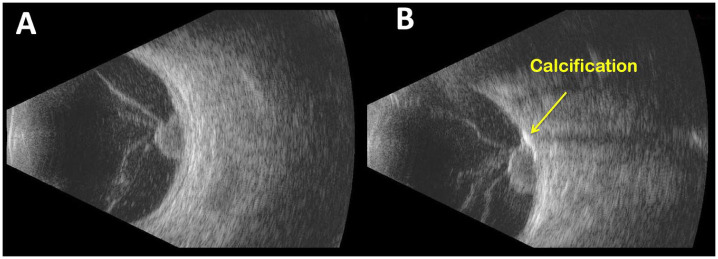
(A) Transverse B-scan ultrasonography of the left eye demonstrating a large, dome-shaped, acoustically solid intraocular mass arising from the posterior pole. The lesion exhibits a characteristic Derby hat configuration. No orbital or extrascleral extension is observed. (B) Magnified view of panel A demonstrating basal calcification associated with posterior acoustic shadow.
